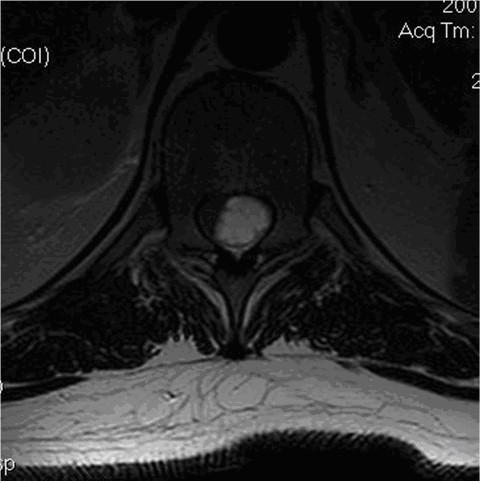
Axial T2-weighted thoracic magnetic resonance image. Note intradural lesion at T10 (arrow).
